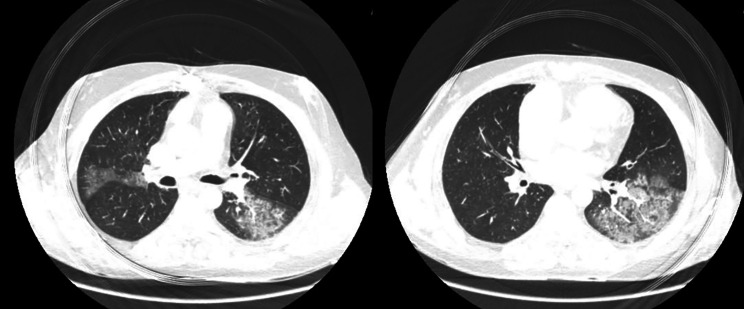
Chest CT scan shows ground glass opacities mostly in left lung in favor of COVID-19 infection
